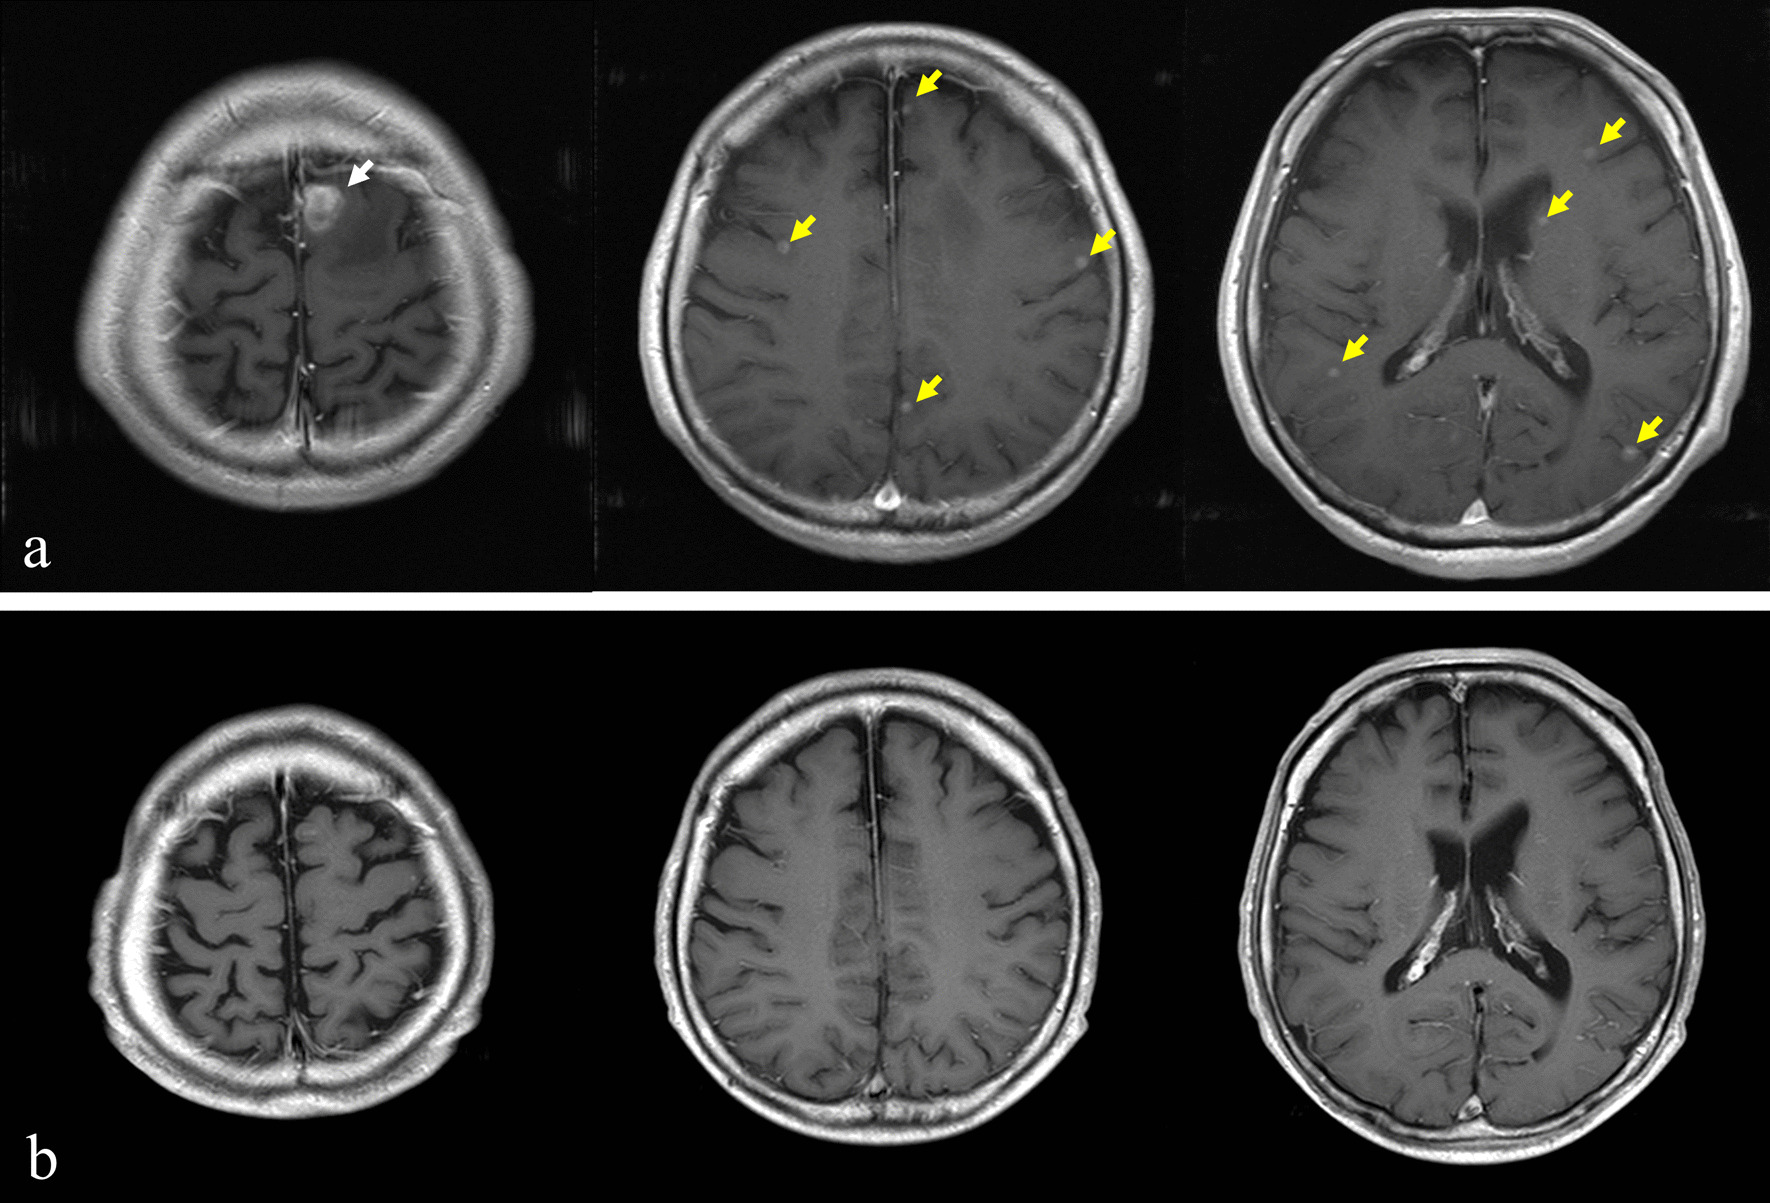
Magnetic resonance imaging showed multiple brain metastatic masses 3 months after radiotherapy for the ninth vertebra (a). The tumor in the frontal lobe was enlarged (white arrow), and numerous other metastases appeared (yellow arrows). Whole-brain radiotherapy (WBRT) was performed 2 months after the initial radiation therapy. After WBRT, all brain metastases disappeared (b)
